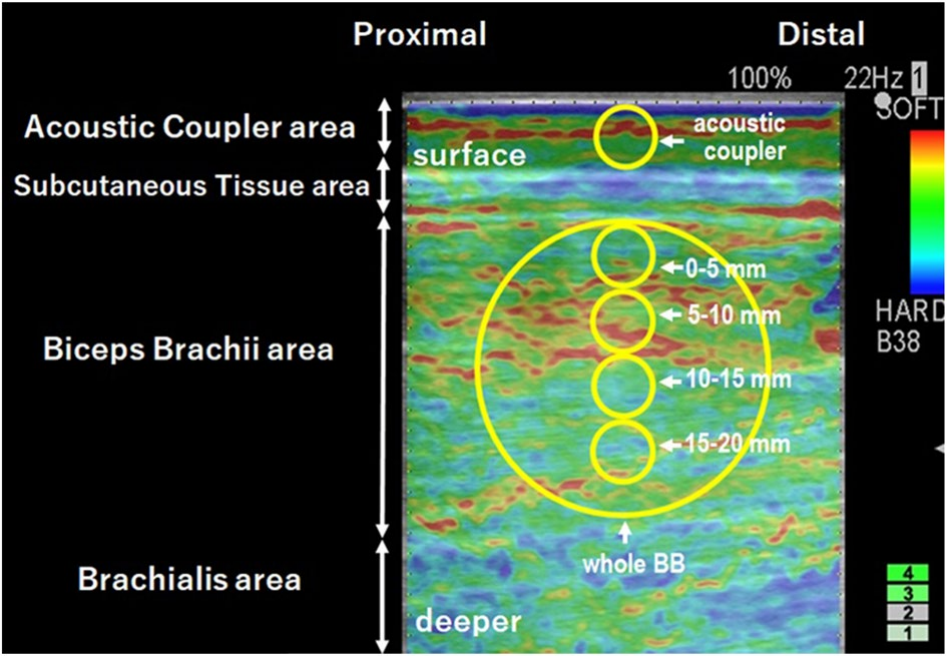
An ultrasound strain elastography image with an acoustic coupler taken from the biceps brachii muscle (BB). The region of interest (ROI) was set for acoustic coupler area (5-mm in diameter) and a circle including the whole BB (large circle) and a circle with a diameter of 5 mm from shallow to deep regions. First to 4th ROIs (0–5 mm, 5–10 mm, 10–15 mm, 15–20 mm) and whole BB were used for analysis, then ROIs deeper than 20 mm were excluded from analysis because of small number of data.
